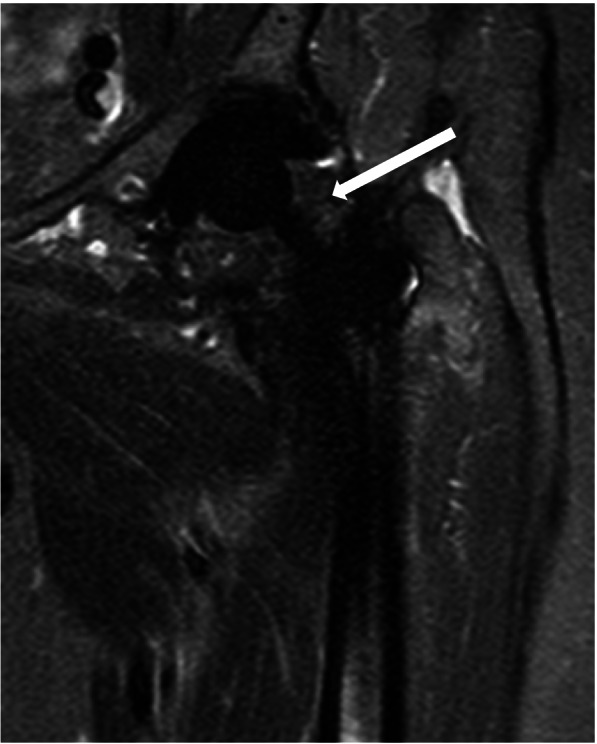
On coronal MARS-STIR-MRI granulomatous synovitis (white arrow) indicates polyethylene wear debris
